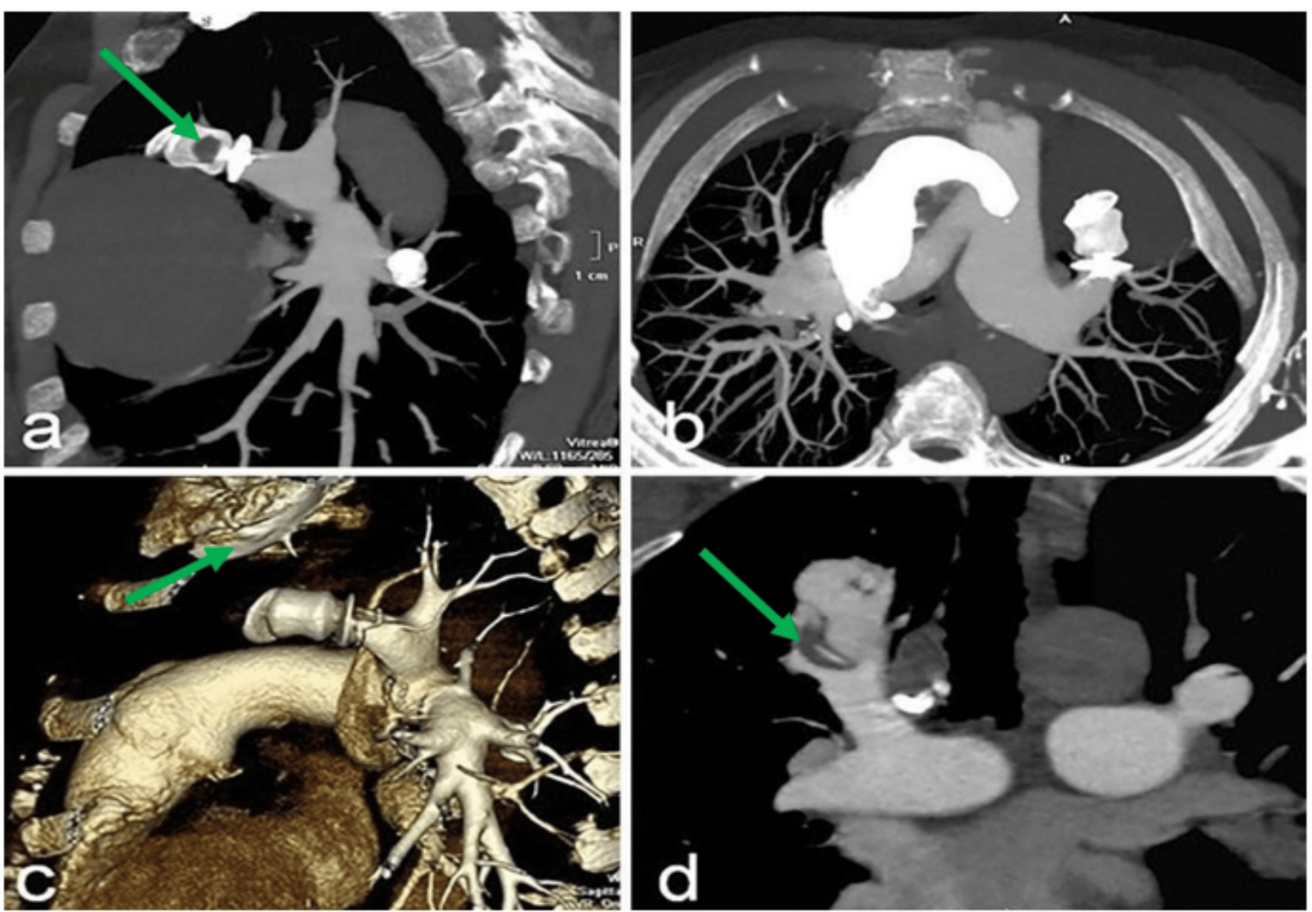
(a-d) Case 2 CTPA post-processing images CTPA (a) sagittal, (b) axial, and (d) coronal MIP images and (c) VR image showing the detailed views of the vascular structures and suspected blockage (green arrow) CTPA: computed tomography pulmonary angiography; MIP: maximum intensity projection; VR: volume rendering
